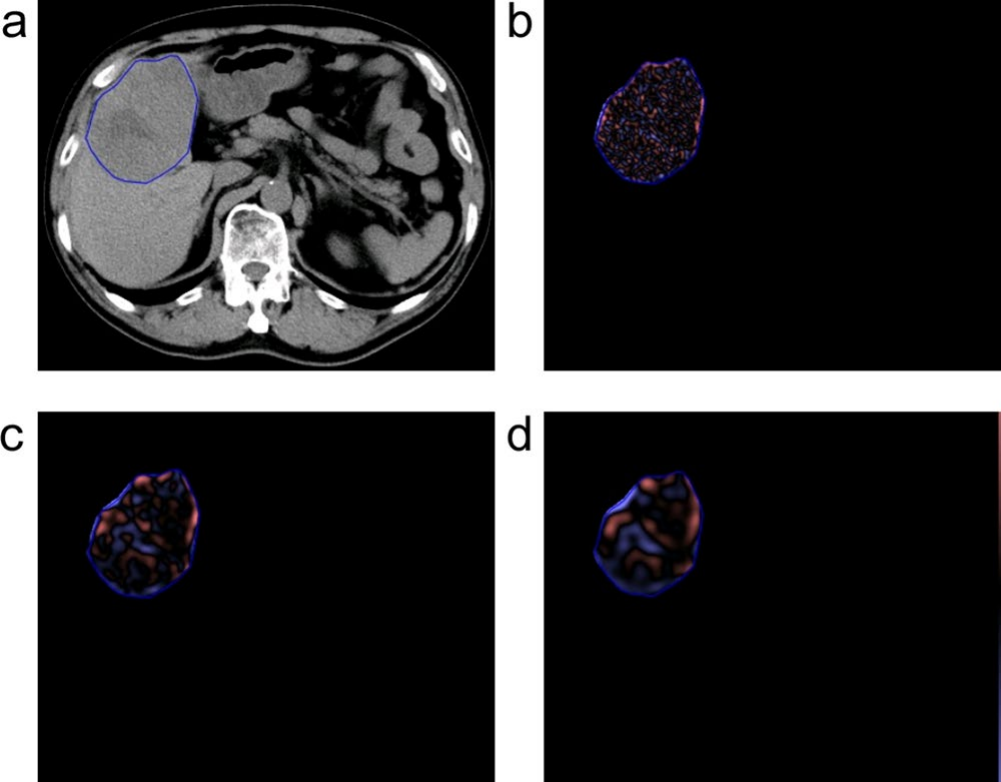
Texture analysis of computed tomography (CT) images of hepatocellular carcinoma (HCC). (a) Preoperative non-contrast-enhanced CT image obtained before hepatic resection shows a region of interest drawn around the periphery of the HCC. A corresponding post-processed CT image displays (b) fine, (c) medium, and (d) coarse textures obtained at fine (2 mm wide), medium (4 mm wide), and coarse (6 mm wide) filtration, respectively. The images are displayed using a red or blue color scale showing negative or positive pixels respectively.
